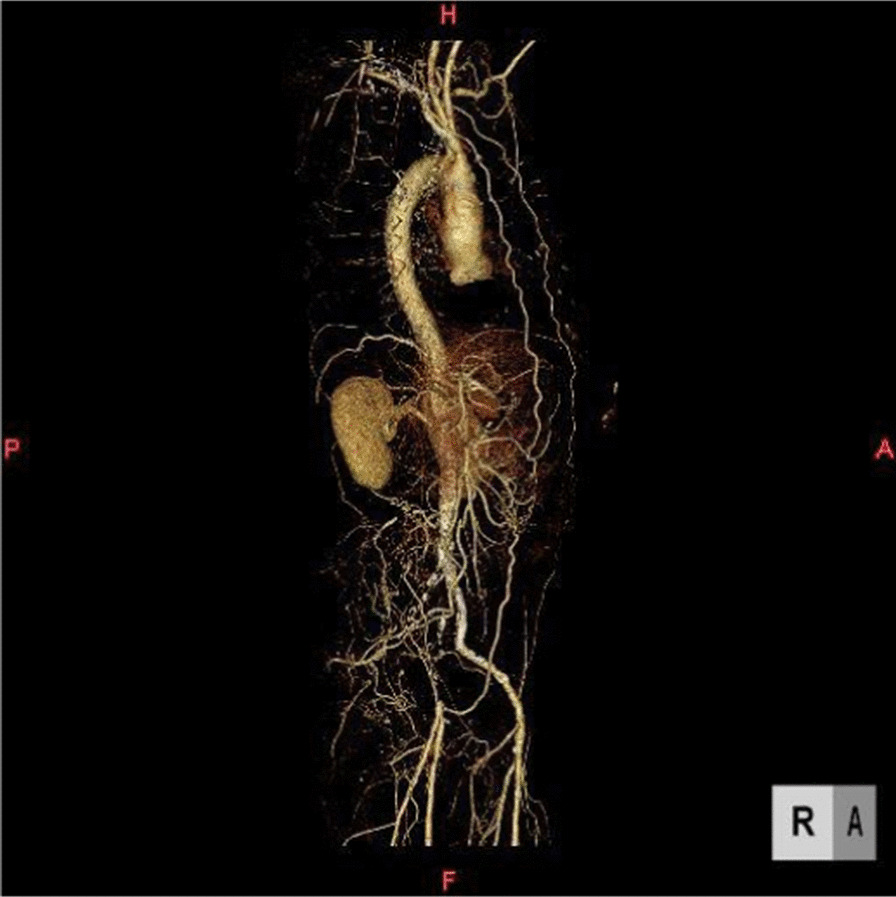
Preoperative computed tomography demonstrated that bilateral internal thoracic arteries are good collateral pathways to the external iliac arteries. Although PTA was performed to the stenosis of the left common iliac artery and the external iliac artery, the PTA to the right common iliac artery was not successful
